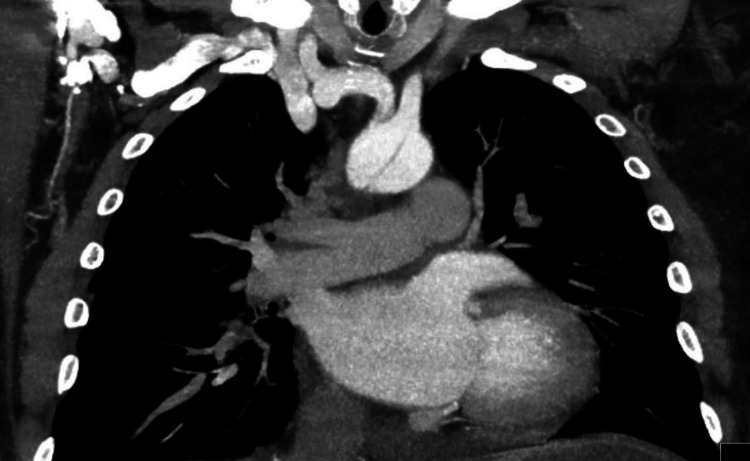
CT angiogram demonstrating aortic arch double lumen
